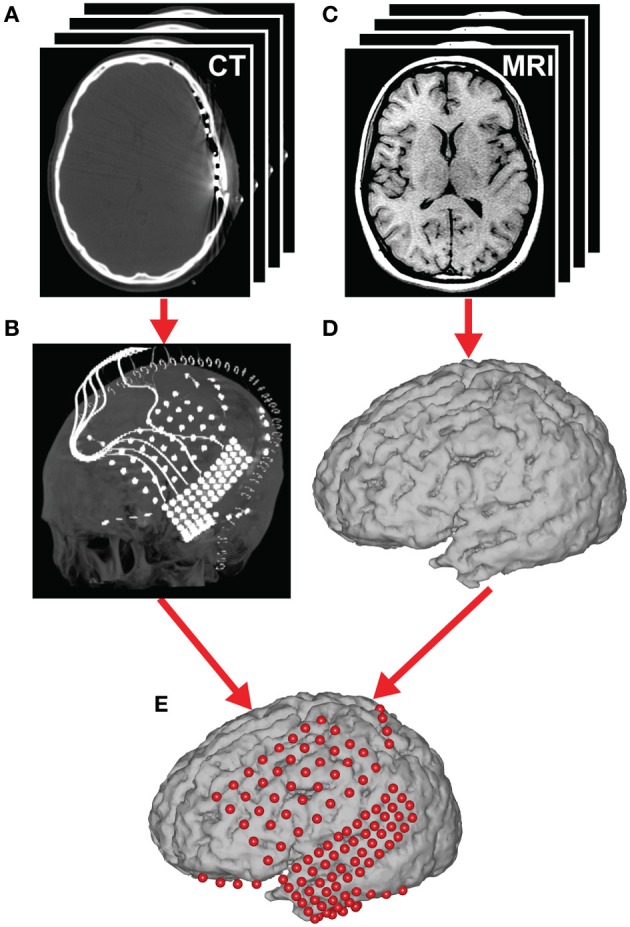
Brain mapping and electrode localization. (A) Post-operative CT scans (1 mm slices) and (C) pre-operative structural MRI scans (1.5 mm slices, T1-weighted) were acquired for each subject. From these scans, grid position (B) and the cortical surface (D) were reconstructed providing a subject-specific anatomical model (E) (see section Coregistration for details).
